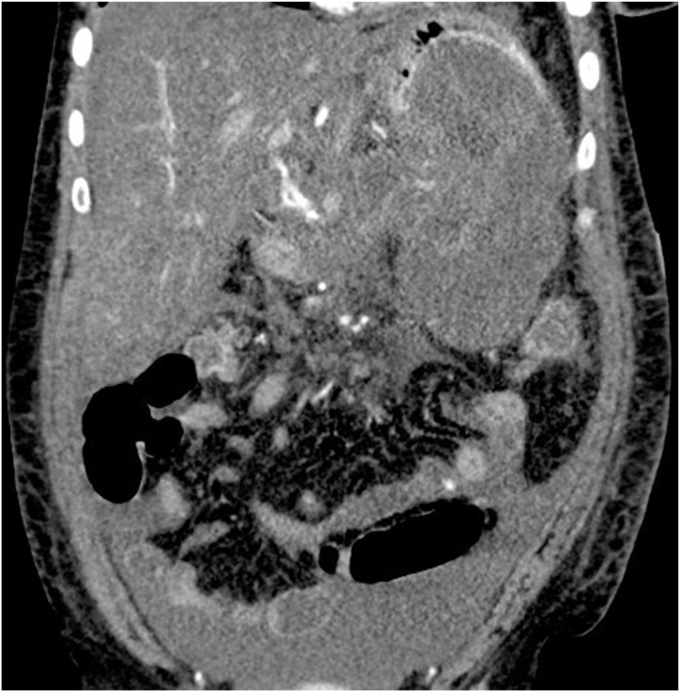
Computed tomography of the abdomen and pelvis showing necrotizing pancreatitis with walled-off collection with debris and small amount of blood products.
